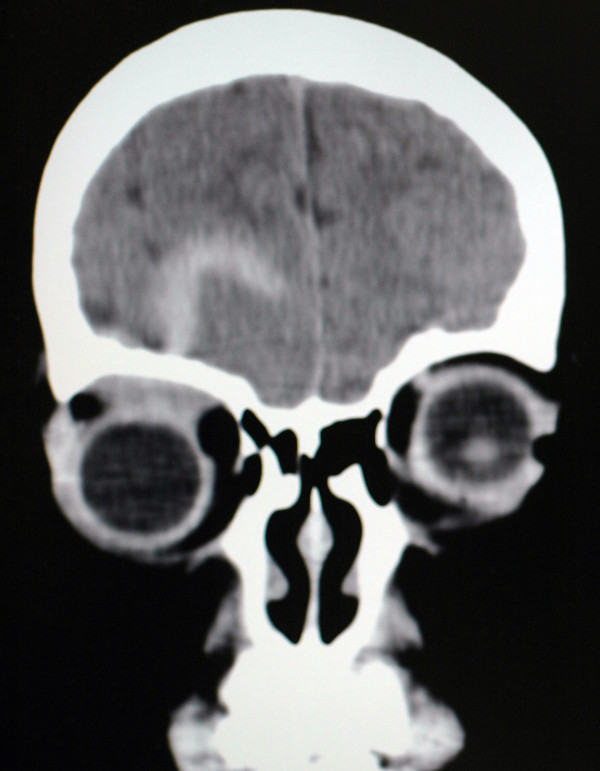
Reconstructed coronal view of a CT image taken at the first visit. A contrast enhanced lesion can be seen within the right frontal lobe area. A low density area is located in the right orbit, just below the upper margin.
